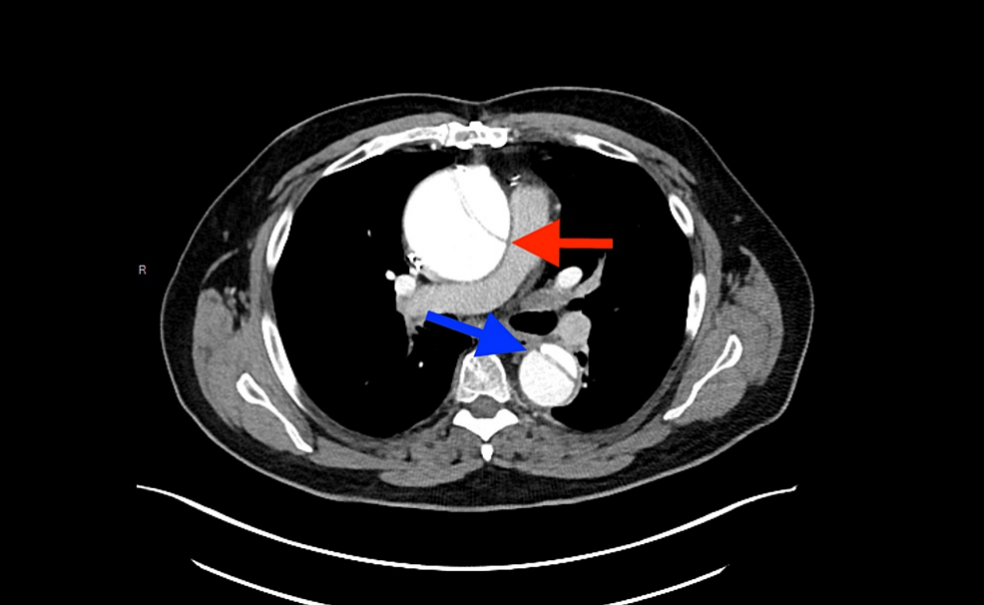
CTA of the chest and abdomen revealing a dissection of the ascending (blue) and descending (red) aorta CTA: computed tomography angiography
